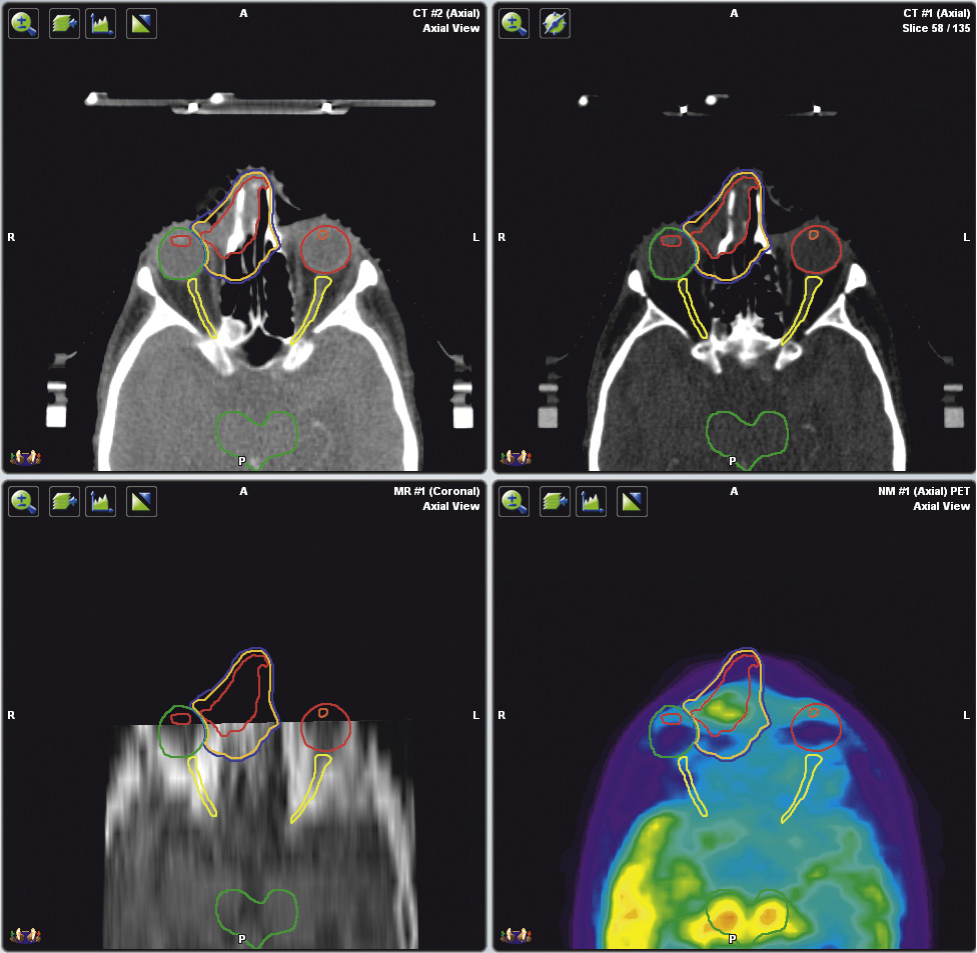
CT, MRI, and PET axial views. Imaging shows the tumor involves the right nasal cavity and medial orbit.
